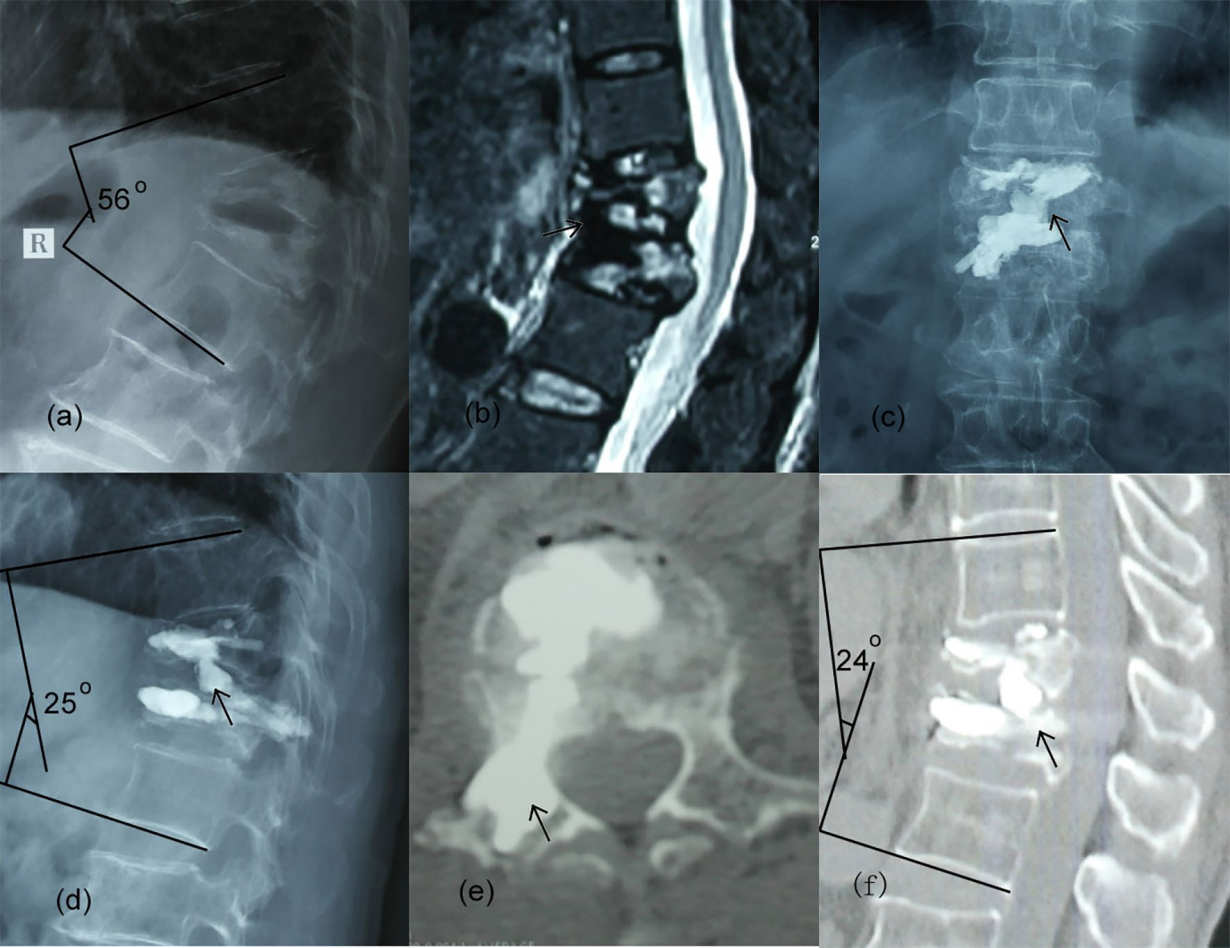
Radiographs of a 73-year-old female with AMOTLFs and EDCI. (a) Preoperative lateral radiograph showed T12–L1 severe fractures with a 50° of thoracolumbar kyphotic angle; (b) preoperative MRI showed subacute T12 and L1 fractures and the EDCI in T12-L1 (arrow); (c) postoperative AP radiograph showed relatively symmetrical cement distribution (arrow); (d) postoperative lateral radiograph showed a cement bridge connected between the two adjacent vertebrae and the kyphotic angle decreased to 25° (arrow); (e) postoperative CT showed cement leakage in the pedicle (arrow); (f) CT reconstruction image in the 2-years follow-up showed the cement bridge was not broke or shifted and the angle sustained well (arrow).
